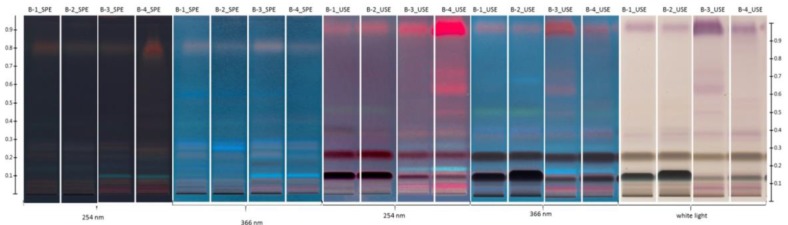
Represented HPTLC fingerprint of buckwheat honey after derivatisation (UAE—ultrasound solvent extraction, SPE—solid phase extraction visualised under white light, 254 nm and 366 nm; B1–B2 cv. Kora; B3–B4 cv. Panda; in Panda cv. we observed additional band on the chromatograms and, moreover, concentration of compound with Rf = 0.13 is less than in Kora cv.
